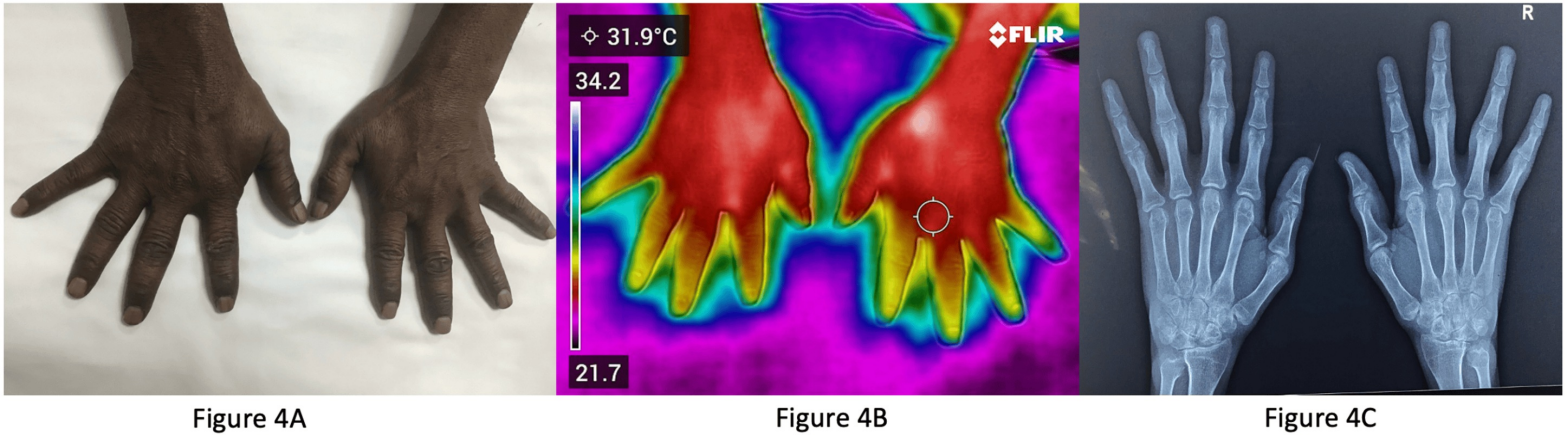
Patient with long-standing rheumatoid arthritis (RA) 4A: Clinical image of another patient with long-standing RA with swelling of the right wrist; 4B: Infrared thermography of the same patient with no increased temperature at the right wrist compared to the other joints; 4C: X-ray wrist of the same patient showing evidence of damage with reduced joint space and erosions.
